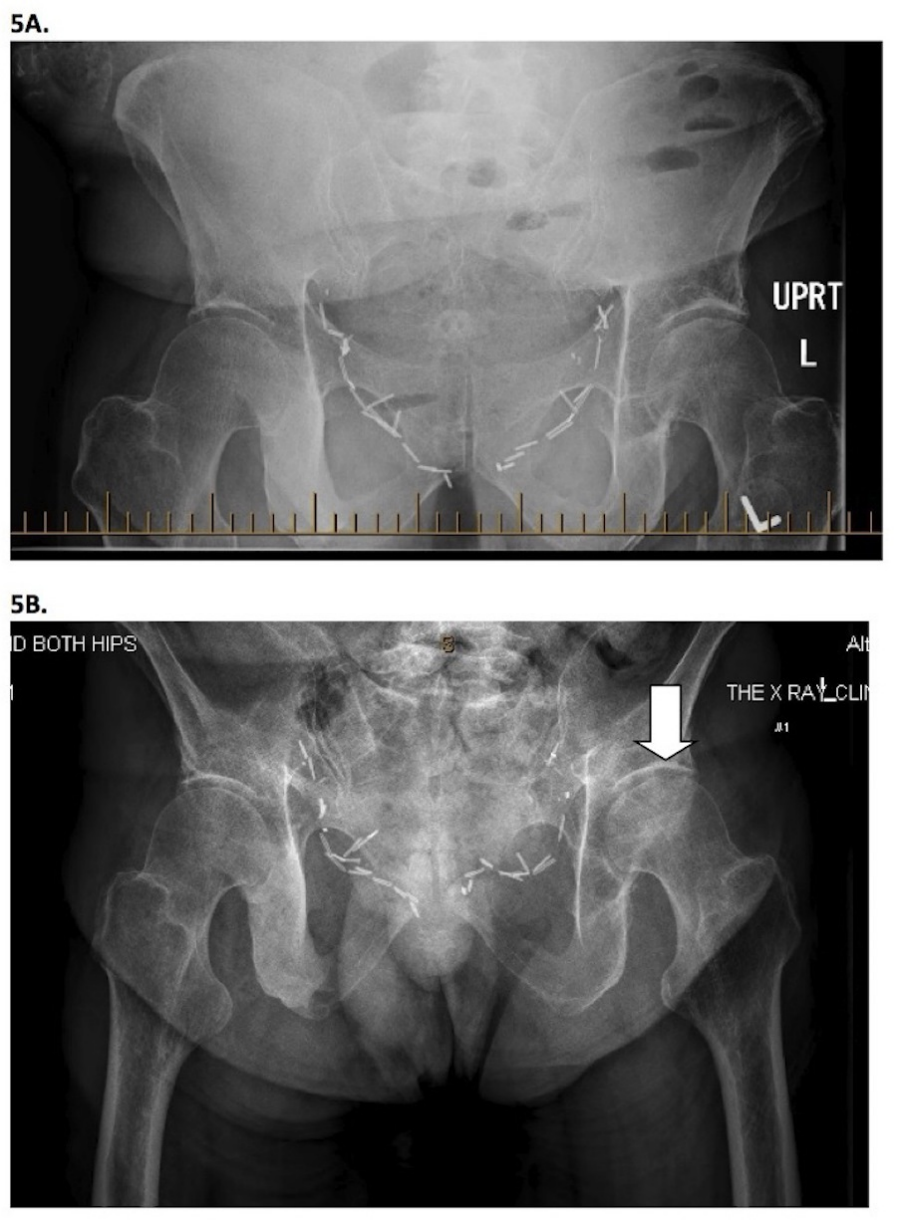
Plain x-rays. 5A: Abdominal plain film (August 11, 2011) demonstrating normal hip joints. 5B: Pelvis plain film (Nov 22, 2011) revealing flattening of the superior articular surface of the left femoral head accompanied by mild juxtaarticular sclerosis and small juxtaarticular cysts (arrow) in keeping with early avascular necrosis.
